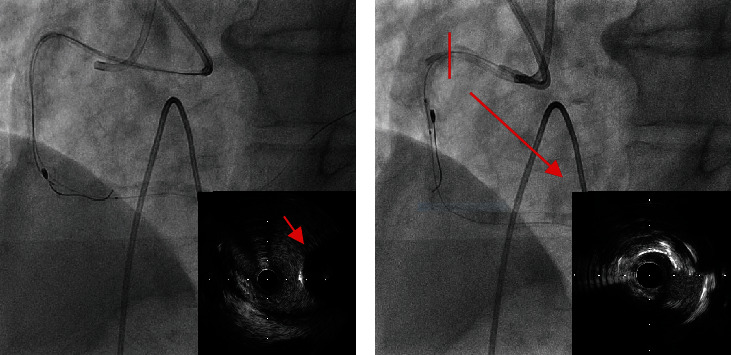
(a) The burr was observed using intravascular ultrasound (IVUS). The burr was not entrapped at this site. (b) Inflated 2.5 mm balloon next to the entrapped burr. The inner side of the proximal lesion of the bending RCA was observed by IVUS to be ablated.
